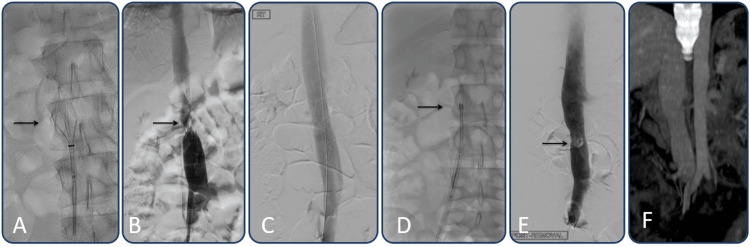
A 23-year-old trauma patient who had an inferior vena cava (IVC) filter placed for prophylaxis prior to orthopedic surgery A) Radiograph shows a partially collapsed Optease filter (arrow) during the initial retrieval attempt. The filter could not be retrieved despite excessive force application. B) Post unsuccessful removal attempt venography showed a high-grade stenosis (arrow) due to IVC collapse during the retrieval procedure. C) Post-balloon angioplasty venography shows patent IVC and resolution of the stenosis. D) On a different session, a 12 Fr GlideLightTM laser sheath (arrow) was introduced, through a 14 Fr outer sheath, and intermittently activated to gradually collapse the filter. E) Post removal venography shows a residual filling defect (arrow) that may represent a fibrin sheath or acute thrombus. F) Coronal reconstruction of a CT scan of the abdomen two months later shows complete resolution of the filling defect with no residual stenosis.
